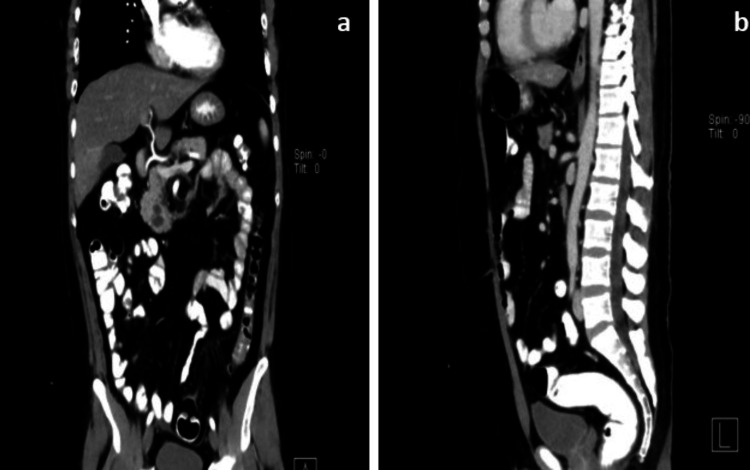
A double-contrast CT scan image. (a) Sagittal section and (b) coronal section, both showing no free air in the abdomen and no intraperitoneal leakage of oral contrast medium. CT: computed tomography
